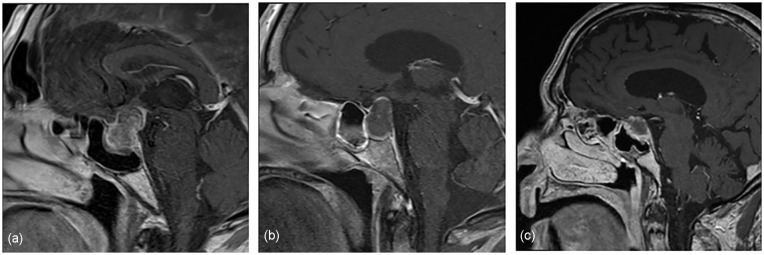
Contrast-enhanced T1WI magnetic resonance images showing (a) irregular internal and peripheral enhancement of the pituitary gland, compatible with a macroadenoma with suprasellar extension, (b) an increase in cephalocaudal dimension of a known macroadenoma with peripheral hemorrhagic contents, and (c) a pituitary gland lesion with intralesional hemorrhagic signals. T1WI, T1-weighted image.
